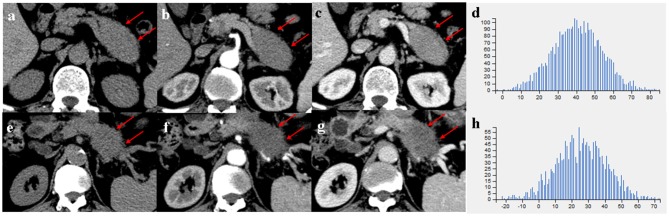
(a–c) A 66-year-old man with MFP, CT imaging showed a pancreatic body tumor (arrows) with a gradual enhancement pattern, the margin of which was well-defined; (e–g) A 68-year-old man with PDAC, CT imaging showed a pancreatic body tumor (arrows) with a hypovascular pattern, the margin of which was ill-defined. (d,h) Histograms of texture parameters of the two lesions showed a marked difference.
